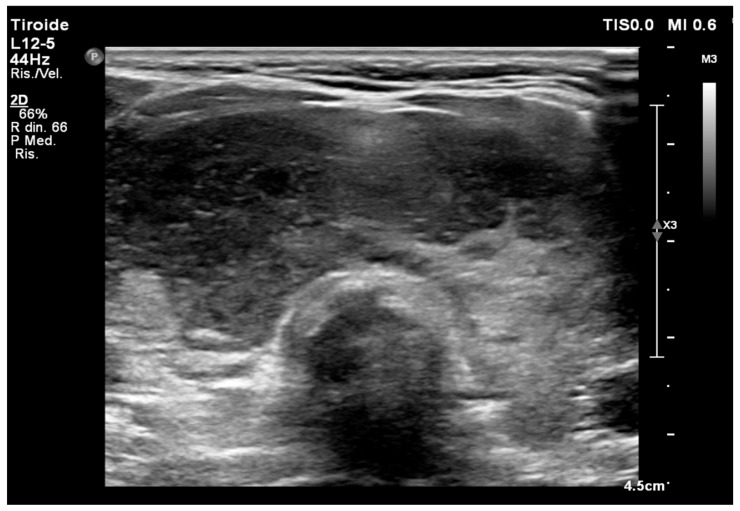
Ultrasound image of one patient of the study series with thyroid lymphoma. It appears as a markedly hypoechoic lesion, with ill-defined margins and absence of calcifications.
